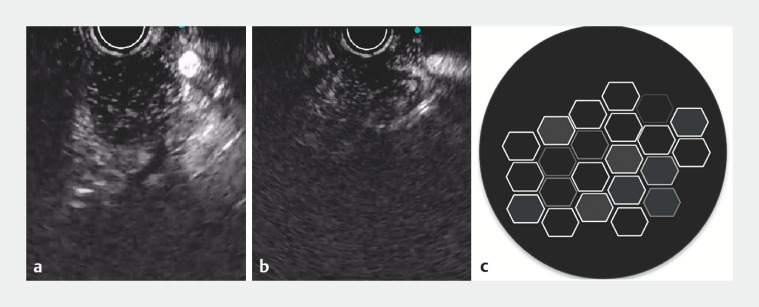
Contrast-enhanced harmonic endoscopic ultrasound revealed hyperechoic solid granular components interspersed with small anechoic regions within the pancreatic mass.
